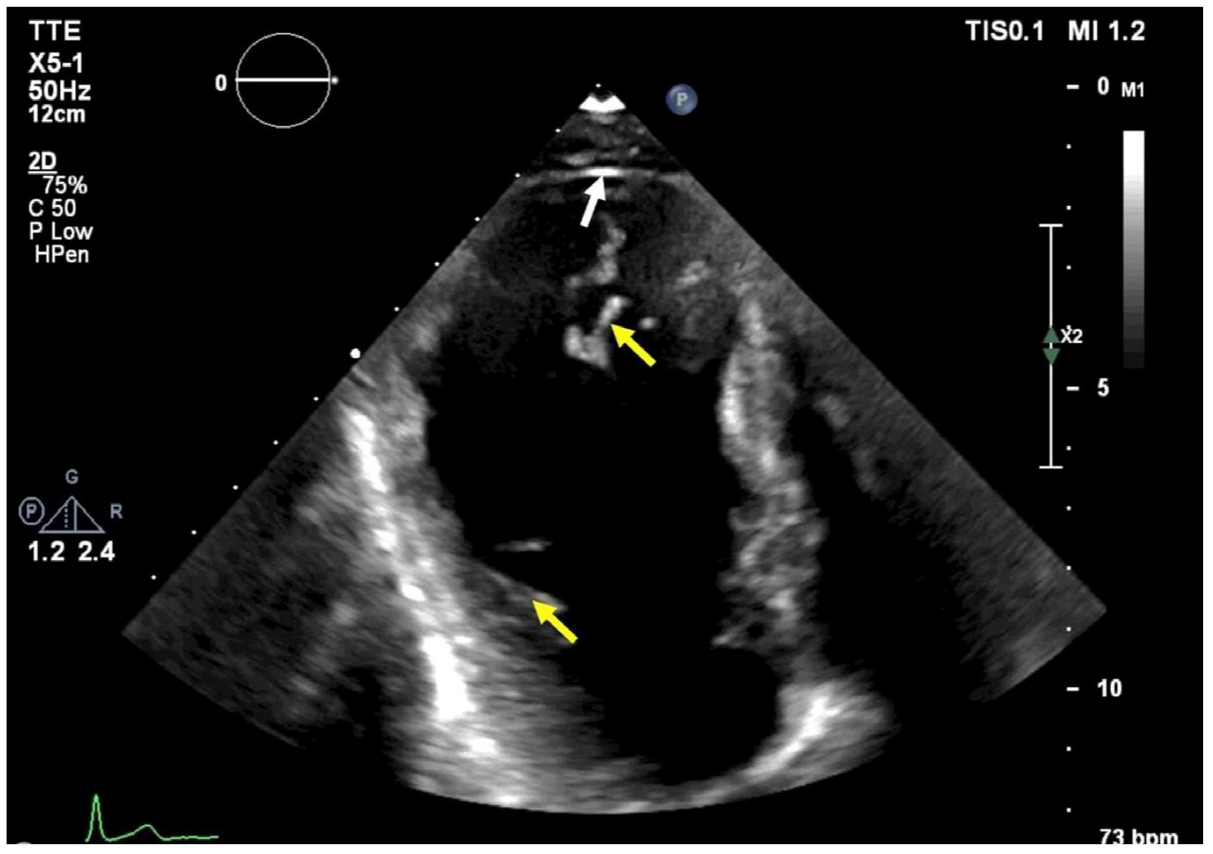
Transthoracic echocardiography demonstrates an isolated apical LV aneurysm (white arrow) associated with trabeculations (yellow arrows).
